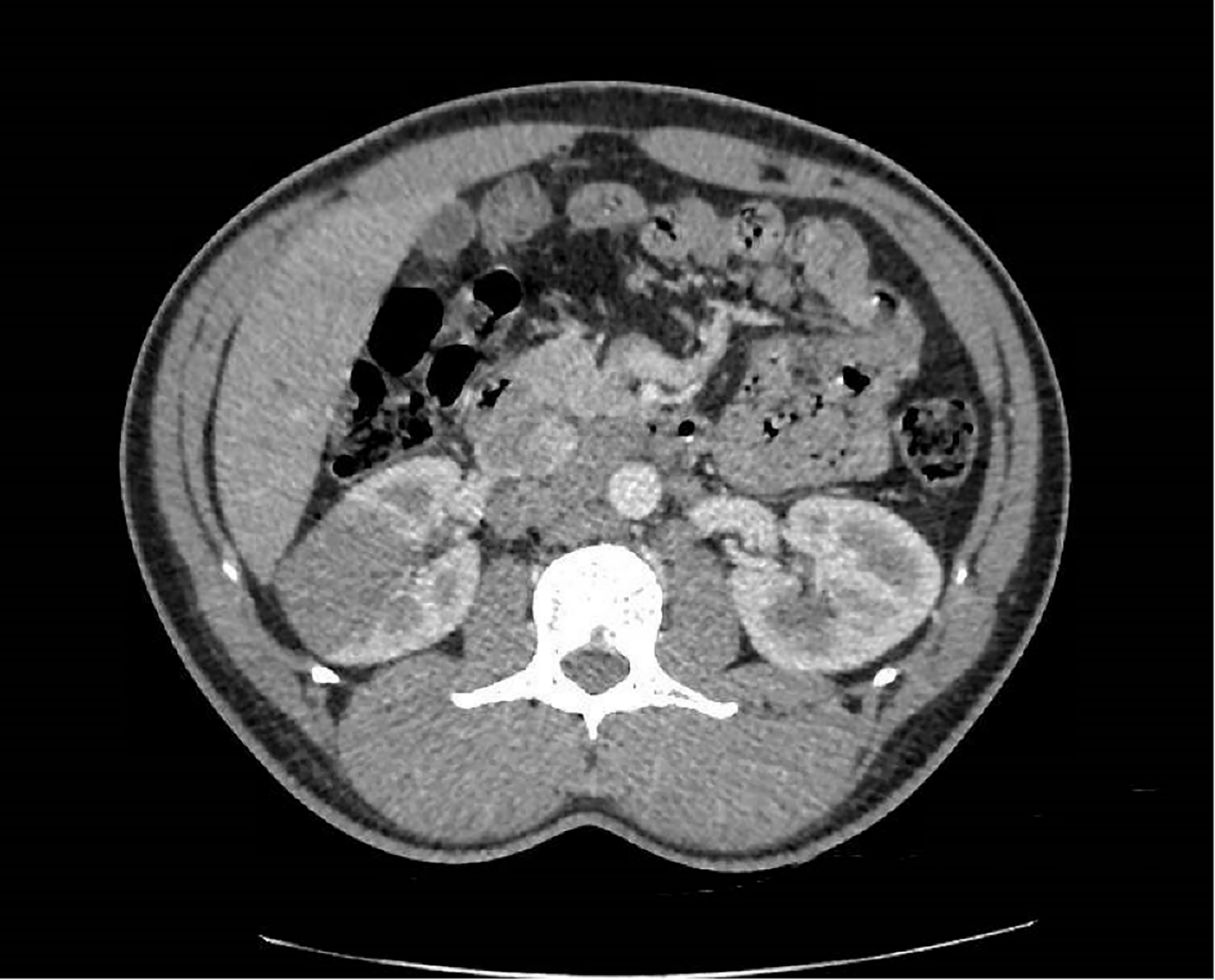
Axial enhanced CT scan showing the poorly delimited right renal mass at diagnosis.
